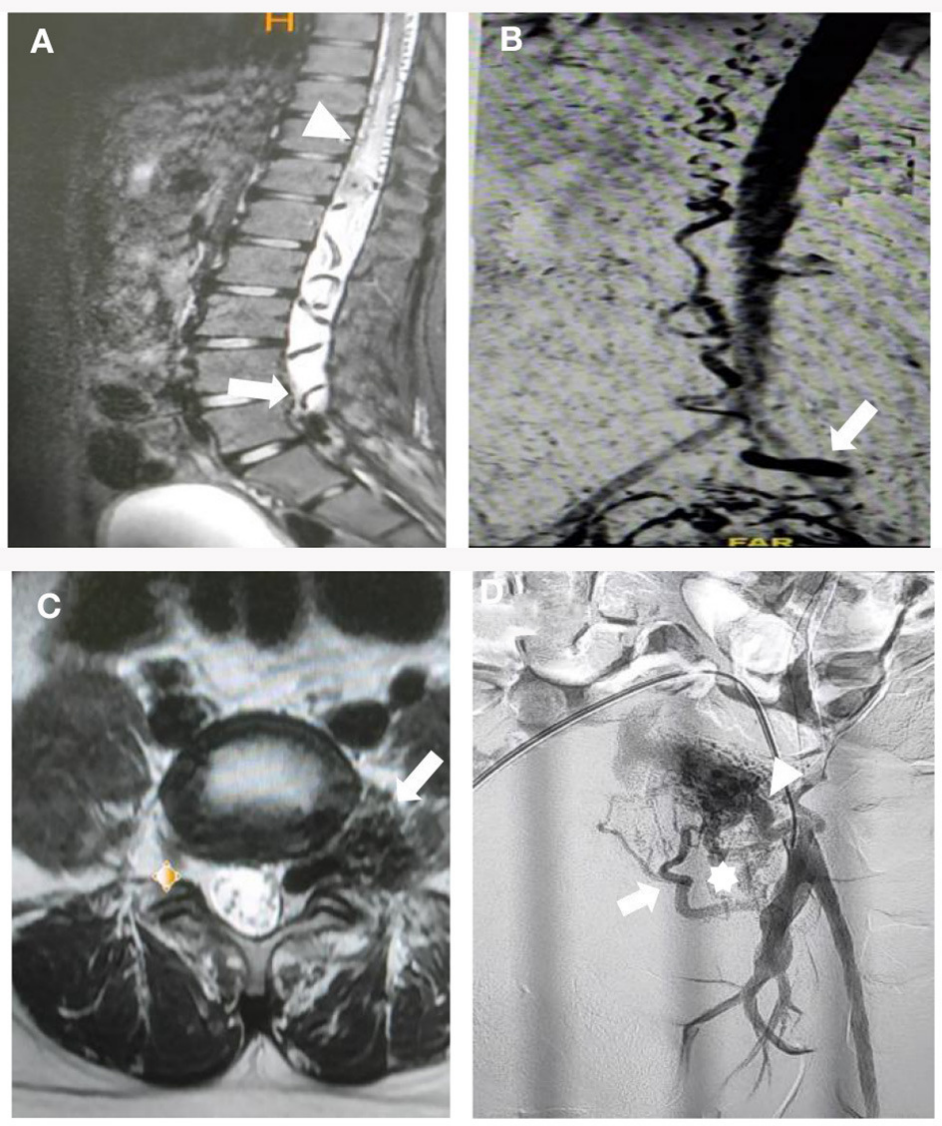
Preoperative multimodal neuroimaging evaluation. (A) Diffuse abnormal central-cord T2-weighted image hyperintensity that suggested spinal cord congestion extending from the level of the thoracic region, seen on magnetic resonance imaging (MRI) with contrast, along with a single dilated flow void that ran from the lumbosacral area to the conus medullaris (arrow). (B) Contrast-enhanced MR angiography (CE-MRA) showed a venous pouch at the lumbosacral region, with drainage cranially from the filum terminale until the cervical level (arrow). (C) Sacral arteriovenous malformation (AVM) was impressed in the left sacral region, with enlargement of the venous pouch before it enters the intradural region (arrow). (D) Oblique projection of angiography from the left internal iliac artery demonstrating that a venous pouch was seen at the left L5-S1 region that was associated with sacral AVM that was fed by hypertrophied lateral sacral arteries (LSA) S1 (asterisk), S2 (arrow), and the sacral branch of the iliolumbal artery (ILA) (arrow head).
